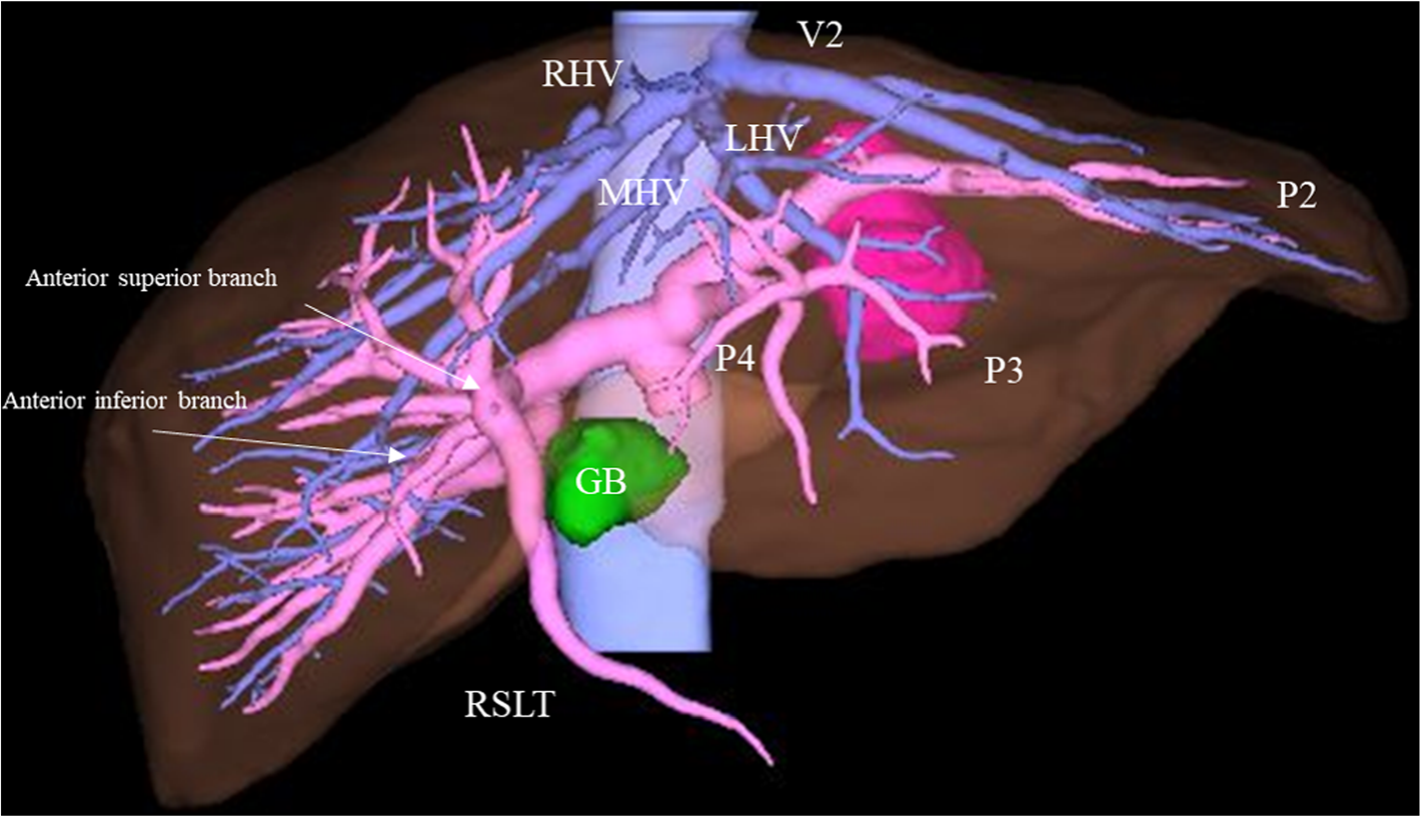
Three-dimensional CT clearly showed that the umbilical portion of the portal vein was located on the right anterior portal vein, where the RSLT connected. P2, portal vein branch of the left lateral superior section; P3, portal vein branch of the left lateral inferior section, P4, left paramedian branch of the portal vein, V2, drainage vein of the lateral superior section; RHV, right hepatic vein; MHV, middle hepatic vein; LHV, left hepatic vein; GB, gallbladder; RSLT, right-sided ligamentum teres
